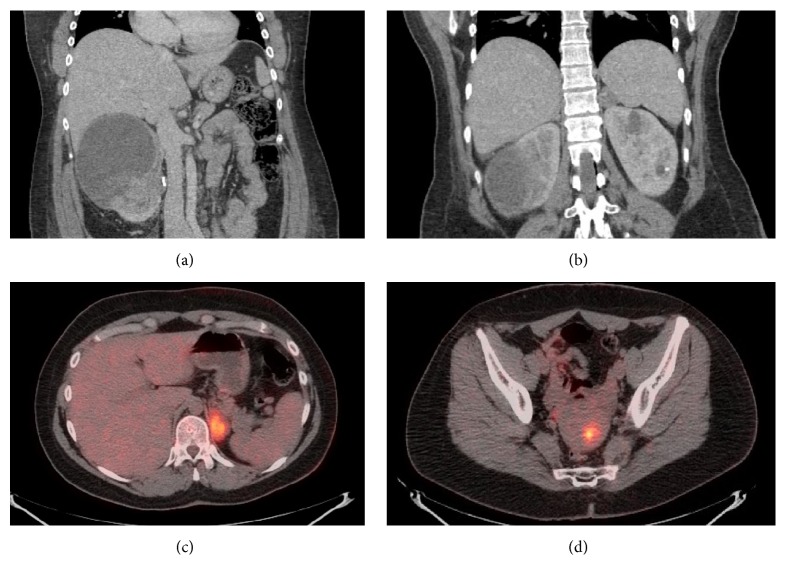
(a) CT representation of the right papillary type 2 renal cell carcinoma. (b) CT representation of multiple cystic structures within the left kidney. (c) PET scan demonstrating FDG-avid left adrenal lesion. (d) PET scan demonstrating FDG-avid uterine focus, likely a leiomyoma.
